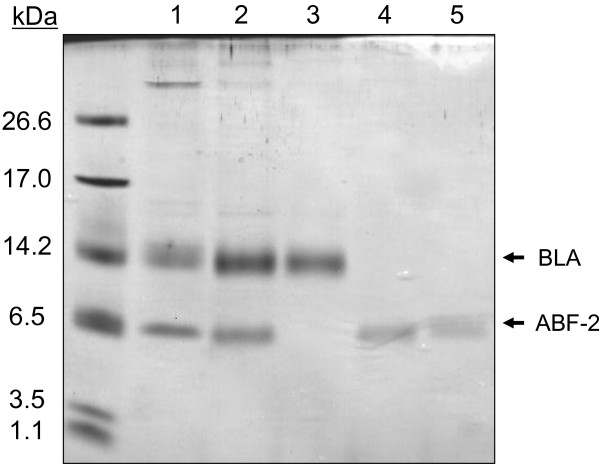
Expression and purification of recombinant ABF-2. Lane 1: Inclusion body after ultrasonication and centrifugation. Lane 2: Solubilized inclusion body. Lane 3: A flowthrough fraction that was passed through cation-exchange chromatography. Lane 4: Purified ABF-2 using cation-exchange chromatography. Lane 5: Purified correctly folded ABF-2 using RP-HPLC.
